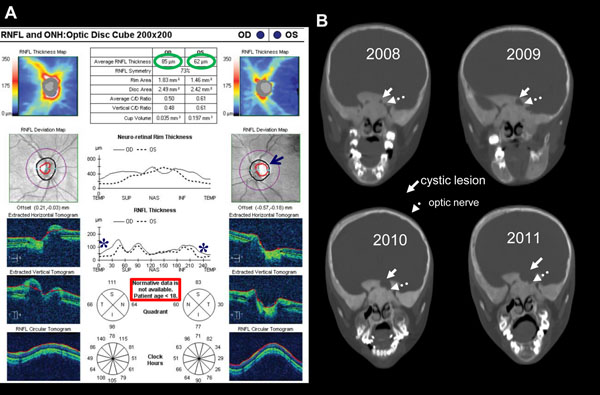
A representative Cirrus^TM^ optical coherence tomography (OCT) optic disc cube study (A) and serial CT scans (B) of a 9-year-old girl with subtle left optic neuropathy and a very slowly expanding cystic lesion abutting the left optic canal. A) The numbers in the two green circles in the RNFL (retinal nerve fiber layer) represent the single number comparison between the two eyes. Generally, the nerve fiber layer is considered thin when it is less than about 70 microns. Note that in the RNFL Deviation Map panels the optic cup (the area within the red circle) on the left (OS) (black arrow) is a bit larger than on the right (OD), also suggestive of axon loss. In the RNFL Thickness graph, note the differences between the left (dashed line) and the right (solid line) in the temporal (TEMP) region (asterisk), indicating that in this region retinal nerve fibers are thinner on the left. For children under 18 normative data for the Extracted Vertical Tomogram and the RNFL Tomogram are not available. B) Serial coronal plane CT images at approximately the same region are shown. The expansile cystic lesion is indicated with the solid white arrow, and the optic nerve by the dashed arrow. The findings indicate the presence of a slowly expanding lesion, the cystic, fluid-filled nature of which was confirmed on MRI. On clinical examination, there were subtle findings of left optic neuropathy in that she performed slightly worse on the Ishihara color test and the Pelli Robson test of contrast sensitivity in her left eye. There was no evidence of an afferent pupil defect. Photos also demonstrated subtle temporal pallor of her left optic disc. There were no objective changes in visual acuity. She has been followed clinically with neuro-ophthalmologic examination approximately every three months to assess for any significant progression, which would be an indication for surgical intervention. The findings on the OCT study confirm the clinical impression of a left optic neuropathy and are particularly useful when visual fields are not obtainable or particularly reliable (usually due to age-related inability to perform the test), as well as an objective measure for longitudinal follow-up. The nerve fiber layer findings on OCT can also be used to predict what visual outcome one might expect after a successful decompression surgery. If one were to find a field defect on examination, but the corresponding optic nerve retinal nerve fiber layer was preserved on OCT testing, it would be reasonable to expect full recovery of vision after surgery. However, if there were nerve fiber layer loss, recovery of vision would be unlikely as the findings most likely represent axons that have died back.
